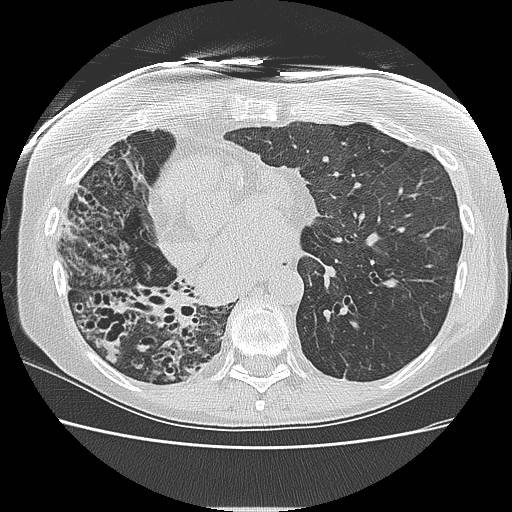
High resolution CT scan of the chest demonstrating bilateral bronchiectasis (right more severe than left) in a patient who underwent right single lung transplantation in 1992 for alpha-1-antitrypsin deficiency and left single lung transplantation in 2003 for bronchiolitis obliterans syndrome.
